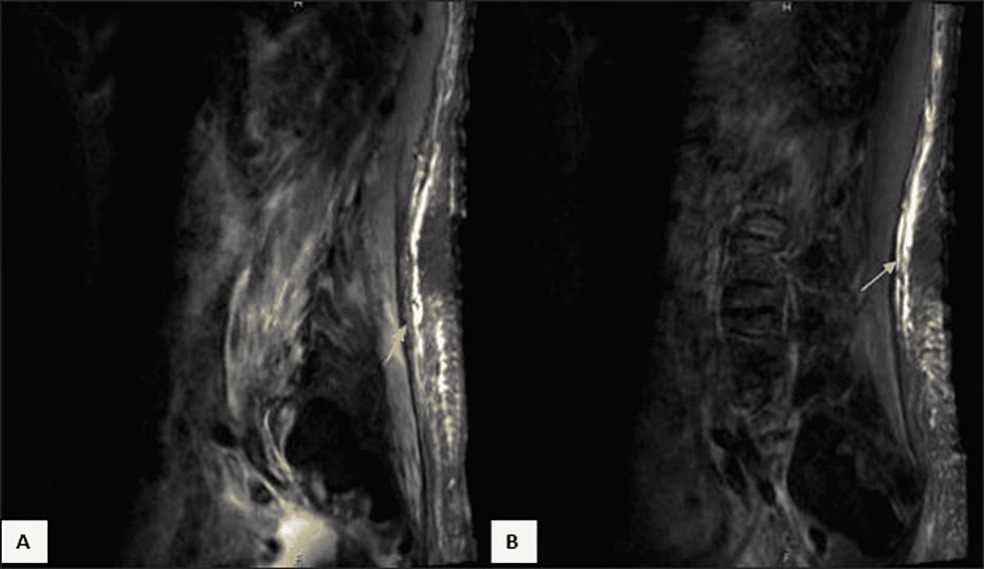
Sagittal STIR weighted image on MRI Sagittal short TI inversion recovery (STIR) weighted image on MRI showing hyperintense signal (arrow) of the right (A) and left (B) paraspinal musculature.
